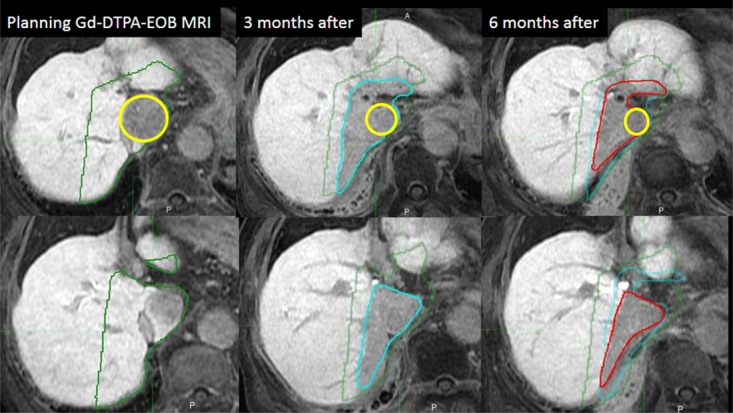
Representative examples of the volume changes of the dFLR or FLR area at planning and on follow-up MRI (upper stage, cranial section; lower stage, caudal section). The dFLR area including the HCC gradually shrank after PBT. dFLR area at planning (252 cm^3^), green; FLR area at 3 months (107 cm^3^), sky blue; FLR area at 6 months (72 cm^3^), red; HCC, yellow. Abbreviations; dFLR: destined focal liver reaction area; FLR: Focal liver reaction; HCC: Hepatocellular carcinoma; PBT: Proton beam therapy; v-TD: visual-threshold dose.
